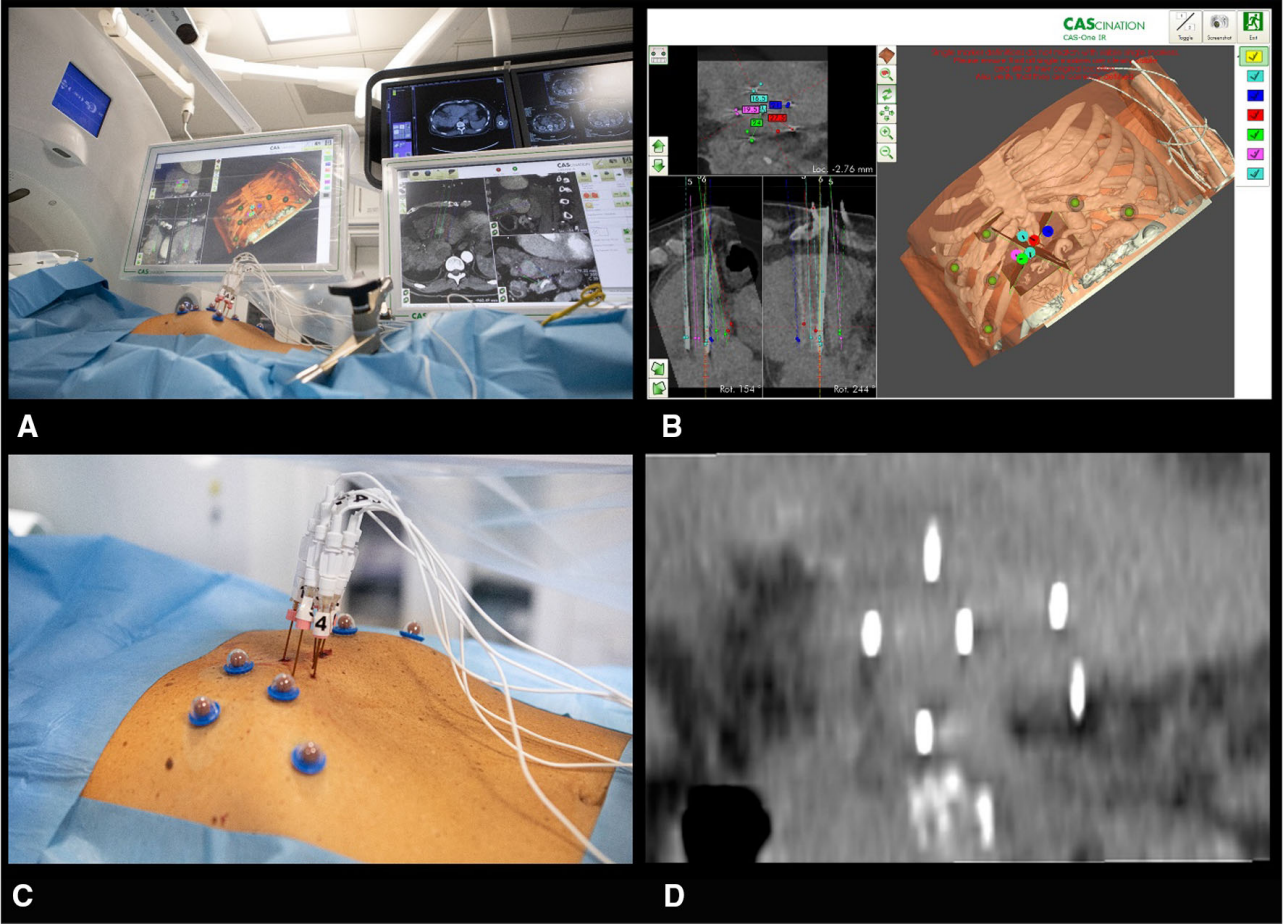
Technical details of the procedure. A Treatment setting in the interventional CT suite using stereotactic navigation (Cascination CAS-ONE IR). B Planning and validation of electrode placement, using the 3D model, generated by the navigation software. C Percutaneous placement of 6 ECT-electrodes (IGEA Cliniporator VITAE). D MPR of the electrode tips in a native control scan demonstrates spacing of the electrode-pairings between 2.1 and 3 cm
